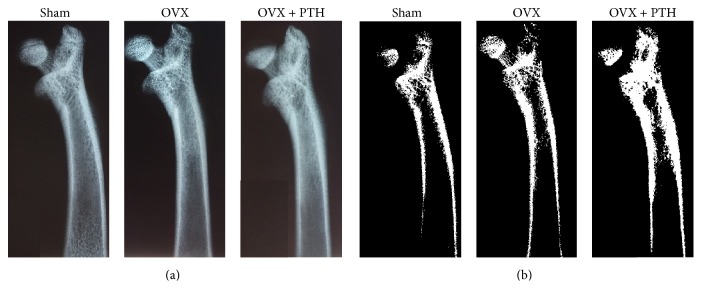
Prevention experiment reference measurements: example of microradiography of Sham, OVX, and OVX + PTH, treated with PTH at 6 weeks after operation. (a) Representative radiographs of femurs bones. (b) Radiographs following threshold analysis. PTH treatment increased the trabecular bone when compared with the Sham and the OVX controls. Slides were conducted with FAXITRON (a cabinet X-ray system, Hewlett-Packard).
